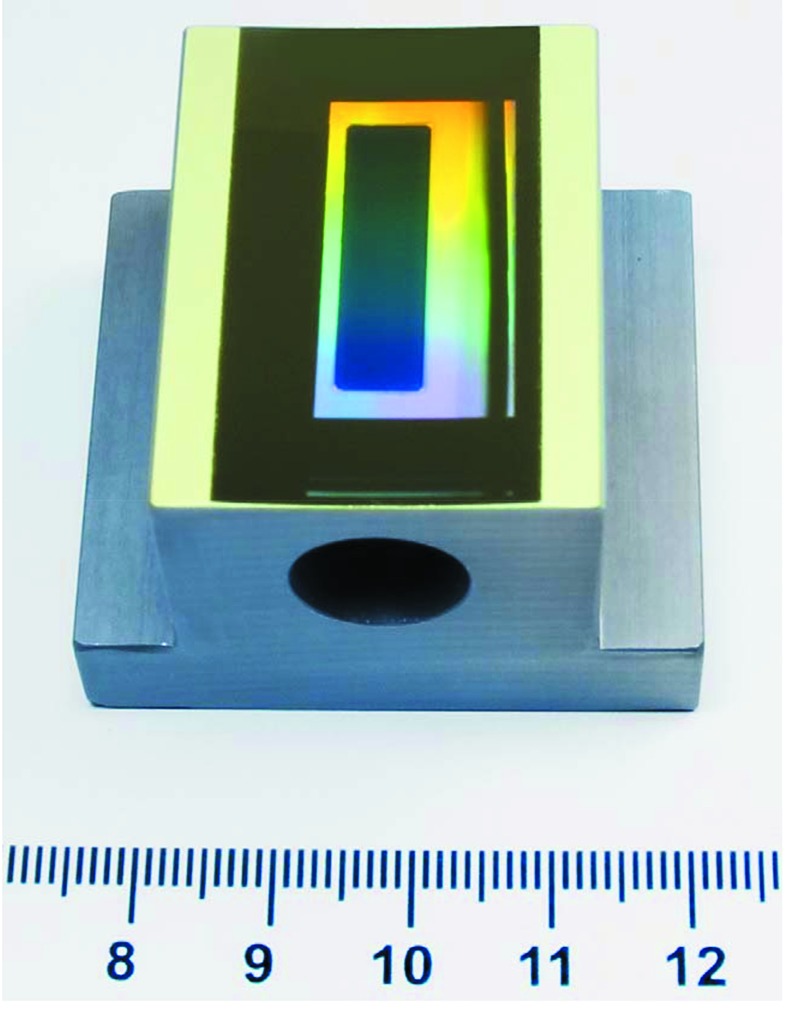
Toroidal VLS grating for soft X-ray self-seeding at LCLS (Stanford). Median line density 1123 lines mm^−1^, blaze angle 1.4°, surface roughness 0.4 nm r.m.s. (with parameters of the toroidal substrate: meridional radius *R* = 195 m, sagittal radius *r* = 0.18 m.
